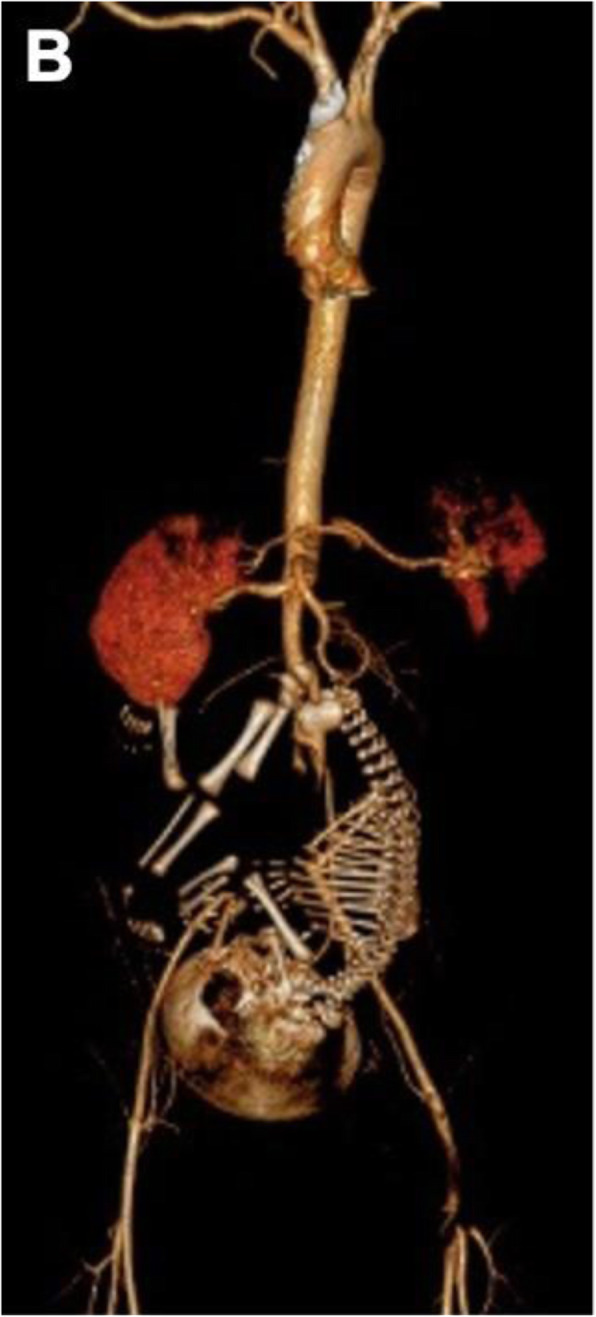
3D reconstruction of CT angiography showing areas of splenic and right renal infarction, thrombosis of the juxta renal aorta and left renal artery, the distal segment of the right deep femoral artery and the left common femoral artery and its bifurcation
